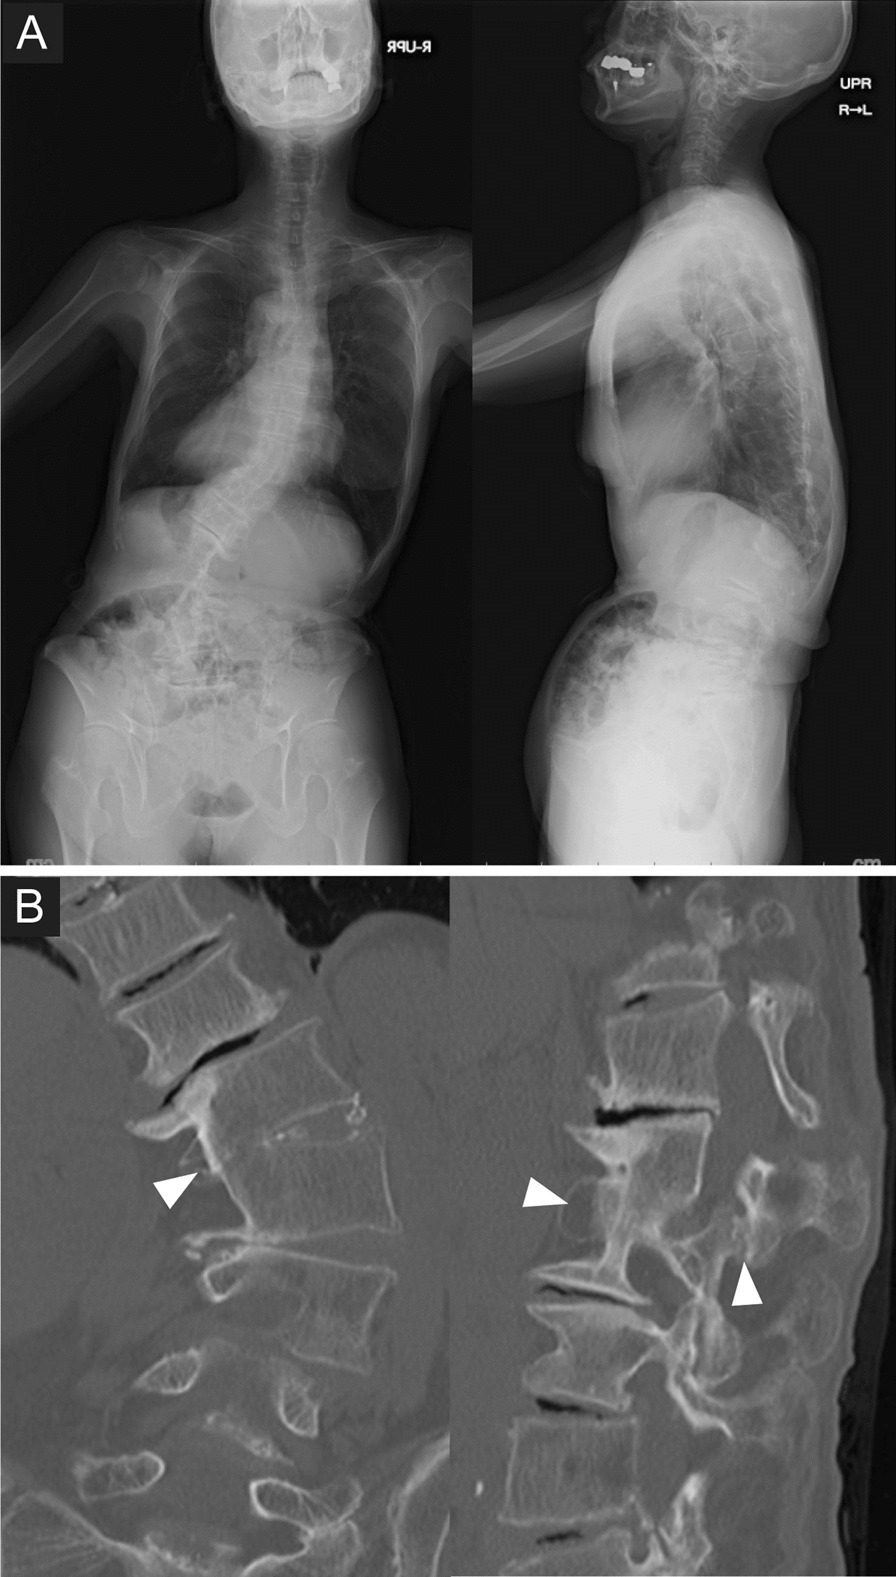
Preoperative images of a 73-year-old woman with rigid kyphoscoliosis treated by two-stage posterior-anterior–posterior surgery. A Preoperative free-standing posteroanterior and lateral spine radiographs. B Preoperative reconstructed CT images. Note that there is partial bony fusion of the L1/2 intervertebral disc and the facet joint (arrowheads)
